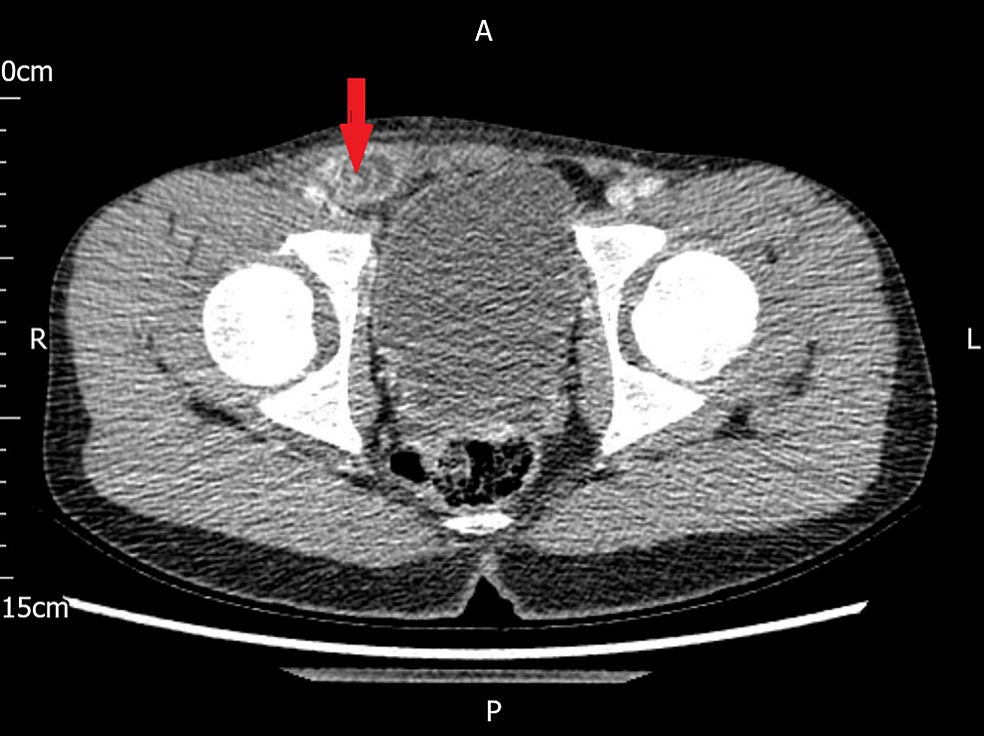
CT axial view showing the right-sided inguinal hernia, containing the appendix.
